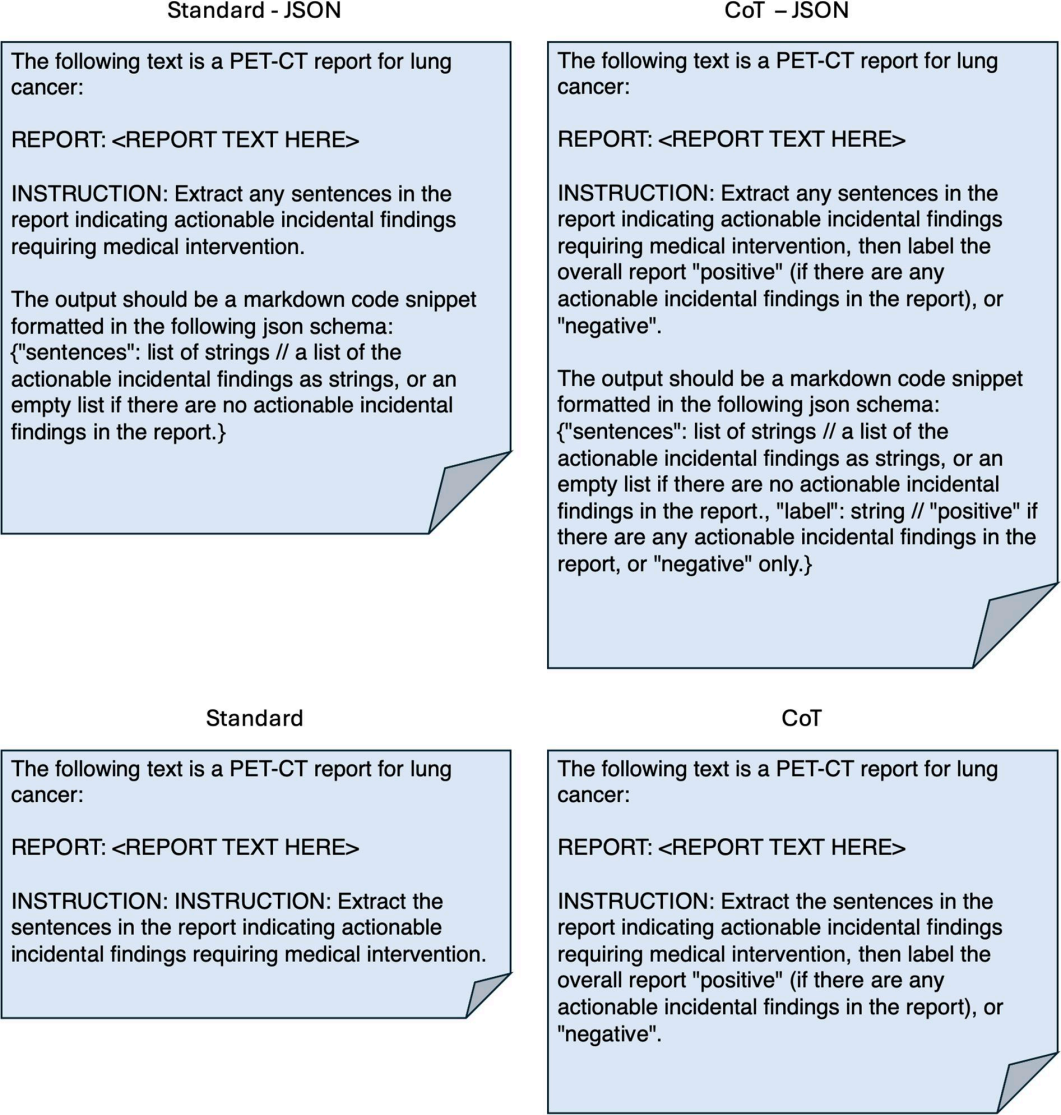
The four prompt templates used for training the model. <REPORT TEXT HERE> represents where the text of each PET-CT report would be inserted into the prompt before tokenization and being inputted to the model.
